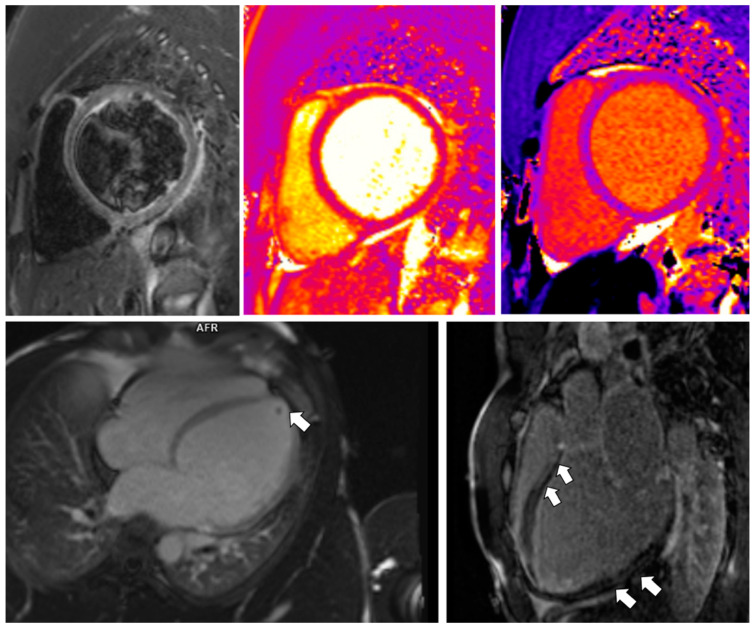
Cardiac magnetic resonance (CMR) findings in Patient 2. Top panel, left: No features of myocardial edema (no significant hyperintensity of the myocardium) can be noted in the T2-weighted short tau inversion recovery (T2STIR) image (ratio myocardial/skeletal muscle signal <2), matched by normal T2 (mid) and T1 (right) relaxation times of the myocardium (see Table 2 for details). Bottom panel, left: A small apical thrombus can be noted in the inversion recovery sequence with a long inversion time (arrow). Right: Subepicardial and intramural streaks of the late gadolinium enhancement (LGE), pointing out areas of predominantly irreversible damage (arrows) in the inversion recovery images, possibly related to healed myocarditis.
